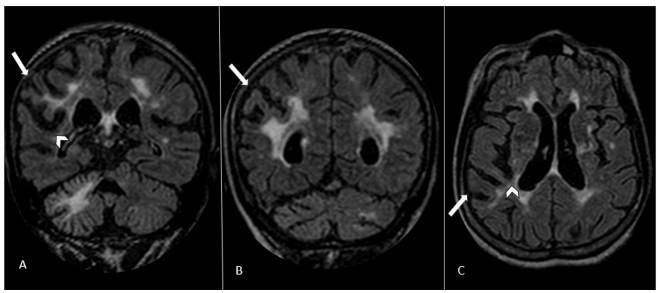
Flair sequences of brain MRI. Coronal (A and B) and axial (C) planes demonstrate asymmetric cerebral atrophy, with a right parietal lobe predominance. The right inferior parietal lobule (full arrow) and homolateral posterior perisylvian region (arrowhead) had a reduced cortical and subcortical volume, most notably in the right angular and supramarginal gyri. Extensive hyperintensity is depicted in the white matter of cerebral hemispheres and right cerebellum, with bilateral ischemic infarction lacunes in nucleocapsular region and cerebral hemispheres, possibly secondary to small vessel disease.
